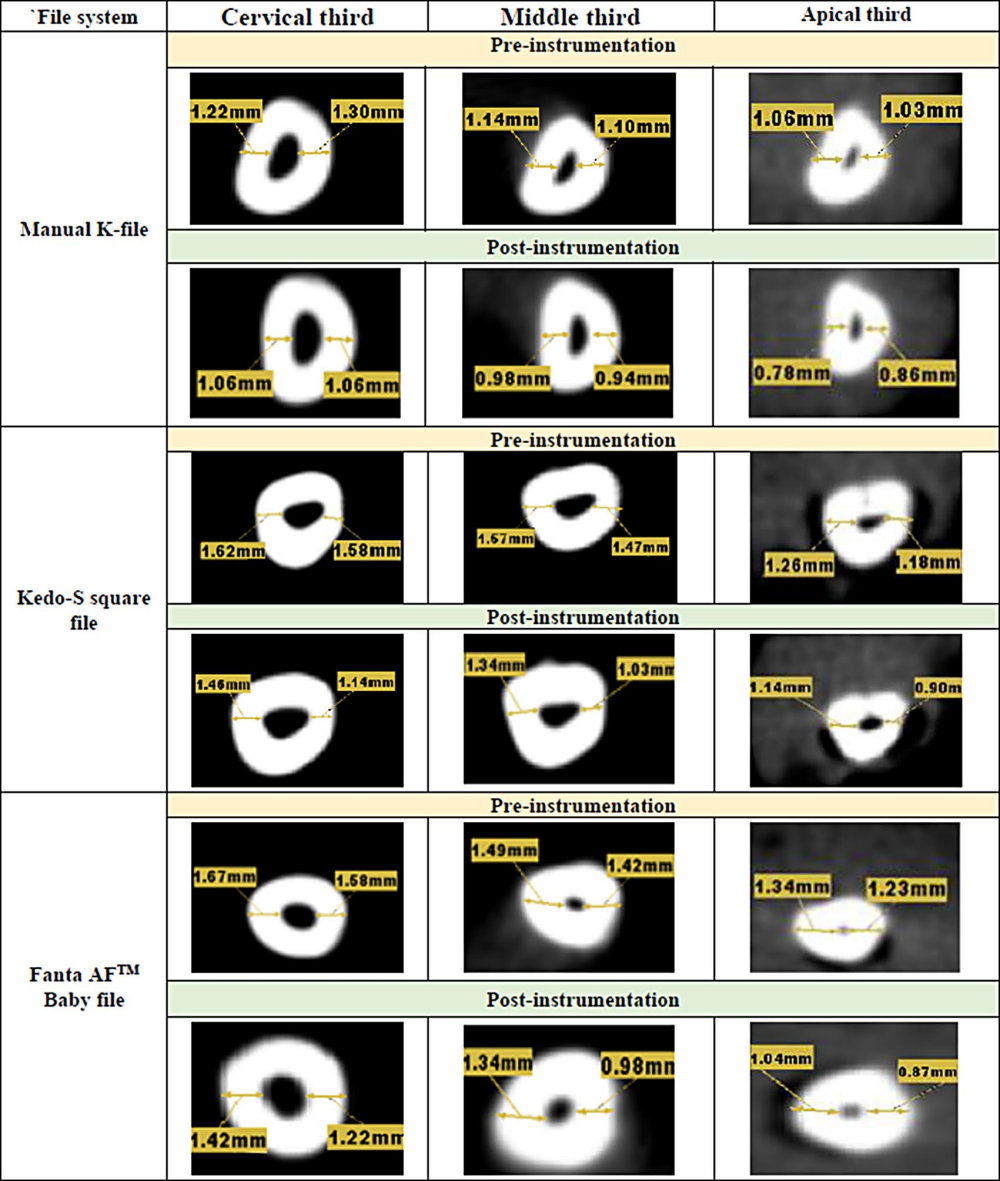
Axial cut of CBCT images showing the amount of dentin thickness pre- and post-instrumentation with manual K-file, Kedo-S Square file, and Fanta AF^™^ Baby file at cervical, middle, and apical thirds
